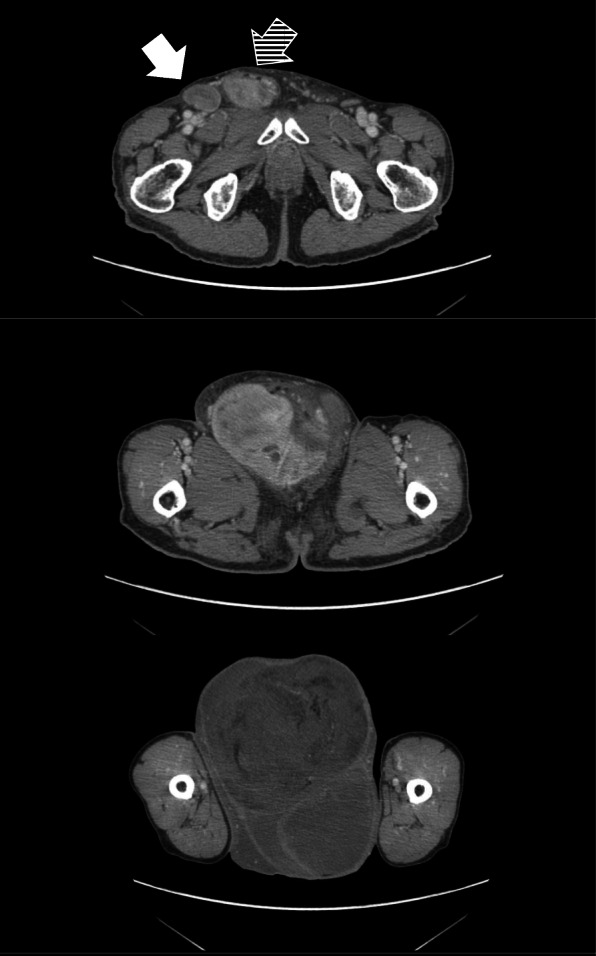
Enhanced computed tomography scan revealed a solid tumor in the right scrotum. The tumor consisted of the enhanced lesion (*middle*) and one with adipose density (*bottom*). Right inguinal lymphadenopathy (*top, white arrow*) and the extended tumor in the inguinal canal (*top, striped arrow*) were observed
